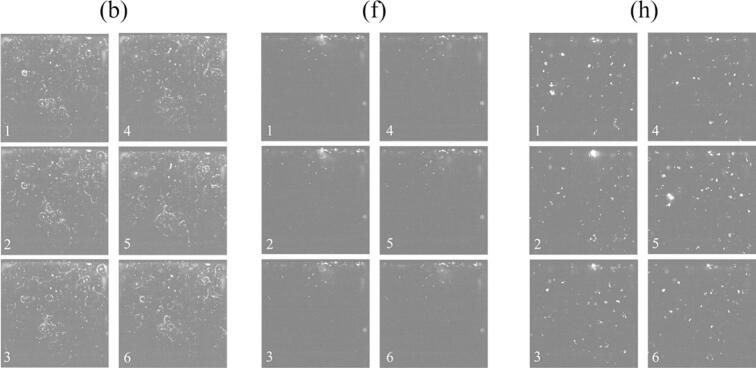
Time variation of bubbles motion and *streamers* formation visualized by green laser in sheet form: with the ultrasonic power of (b)33 W, (f)84 W, (h)136 W. (Supplemental Video 3).
